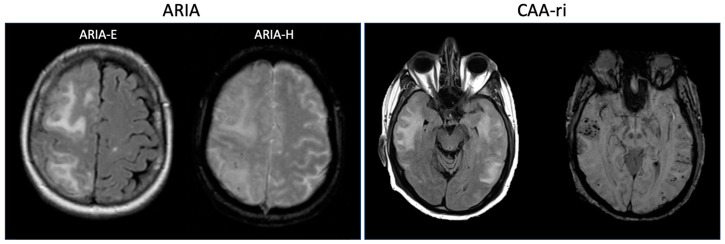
(Left): Representative images from a patient with concomitant ARIA-E and ARIA-H (reproduced with permission from Sperling et al., 2011 [21]). The T2-FLAIR image on the left demonstrates regions of edema (ARIA-E) in the right frontal and parietal lobes, and the GRE image on the right demonstrates microhemorrhages (ARIA-H) in the right parietal lobe. (Right): Representative images from a patient with CAA-ri. The T2-FLAIR image on the left demonstrates edema in bilateral temporal lobes, and the SWI image on the right demonstrates multiple microhemorrhages within these regions.
